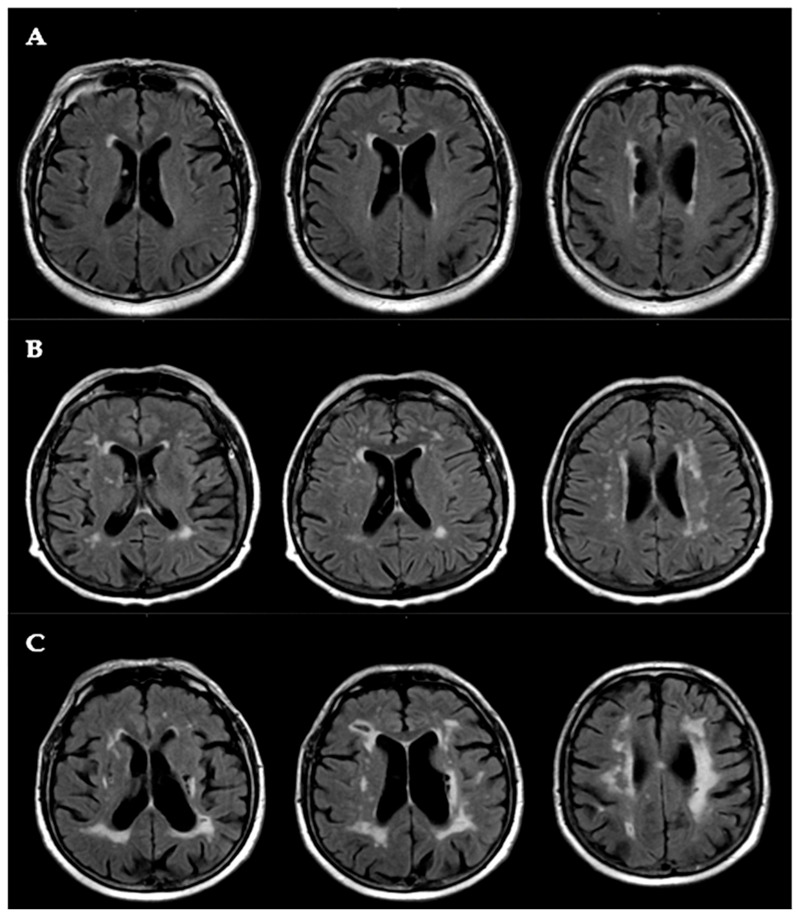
Representative axial fluid-attenuated inversion recovery (FLAIR) MRI images showing varying degrees of white matter lesion (WML) severity, graded according to the Fazekas scale. (A) Mild WMLs: punctate hyperintensities; (B) moderate WMLs: beginning confluence of lesions; (C) severe WMLs: large confluent areas extending into subcortical regions.
